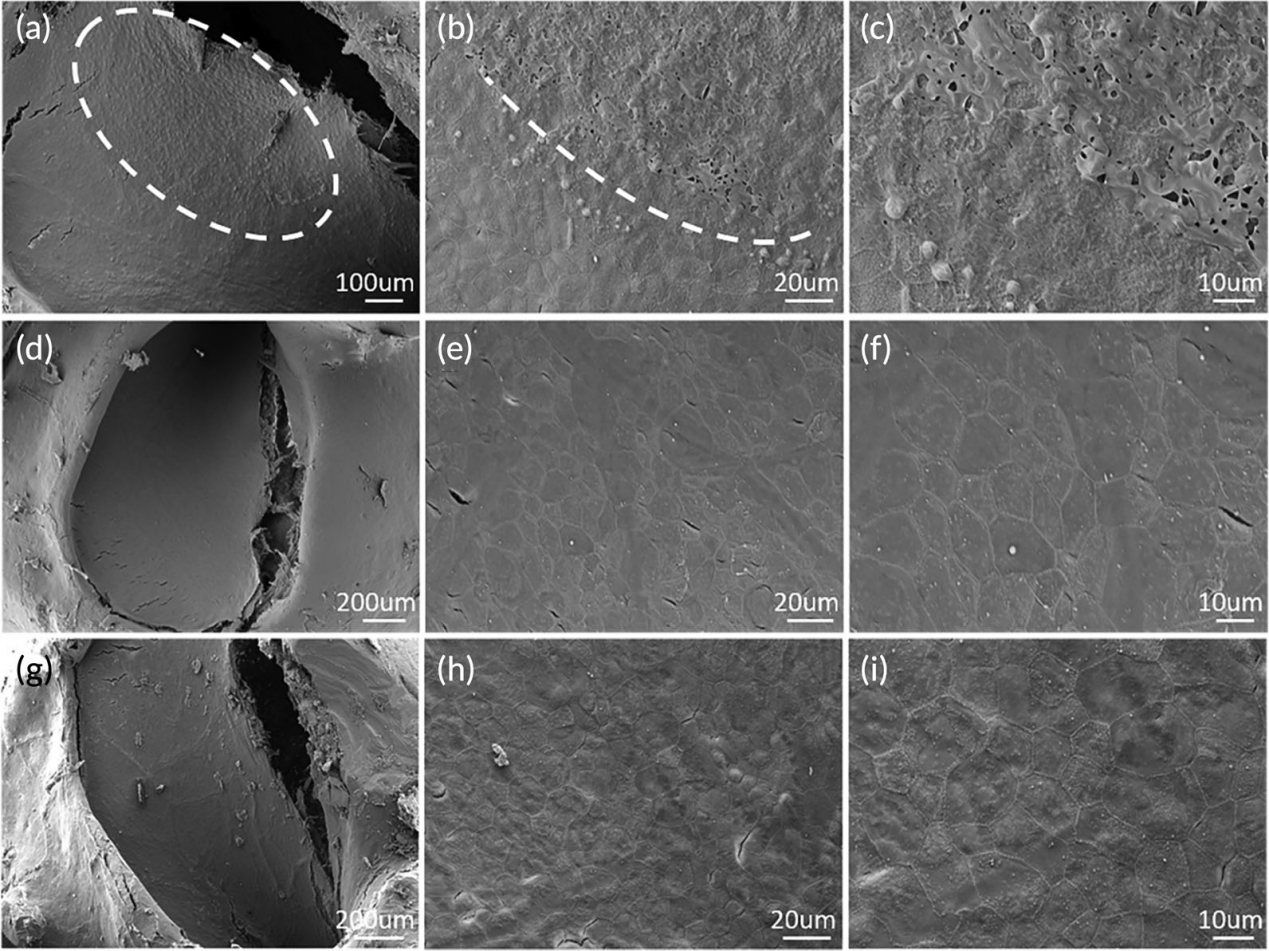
SEM images of the RWM observed at different time points after USMB treatment and the RWM with ultrasound‐only treatment. (a)–(c) 1 day post USMB treatment. The damaged region was circled in (a) and bordered in B by dashed line. (d)–(f) RWM 1 week post USMB treatment. (g–i) RWM observed immediately after ultrasound‐only treatment
